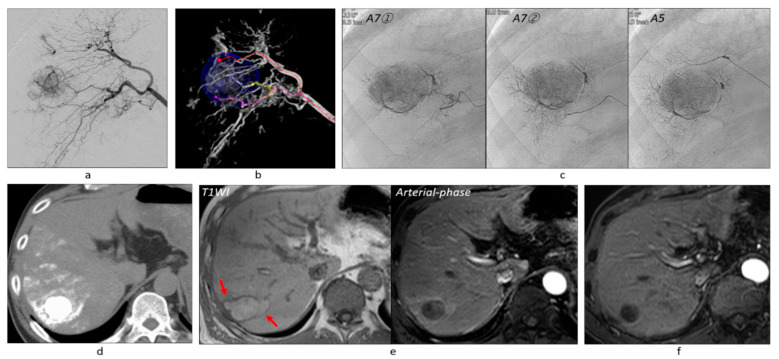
Massive peritumoal necrosis by ultraselective cTACE. (a) Right hepatic arteriogram showed a tumor of 30 mm in diameter. (b) AFD identified three tumor feeders arising from A7 and A5. (c) The tumor feeders were subsequently embolized and Grade 2 portal vein visualization could be achieved. (d) Unenhanced CT performed 1 week after TACE showed dense iodized oil accumulation in the tumor and surrounding liver. Some iodized oil was widely distributed in the anterior segment of the right hepatic lobe via the portal vein. (e) On T1-weighted MRI performed 2 months after TACE, the tumor and surrounding liver showed hyperintensity, indicating coagulation necrosis (arrows). The tumor showed complete necrosis on the arterial-phase of gadoxetate disodium-enhanced MRI. (f) Arterial-phase of gadoxetate disodium-enhanced MRI performed 8 years and 10 months after TACE also showed complete tumor necrosis. Abbreviations: A5, anterior inferior subsegmental artery of the right hepatic artery; A7, posterior superior subsegmental artery of the right hepatic artery; TWI, T1-weighted image.
